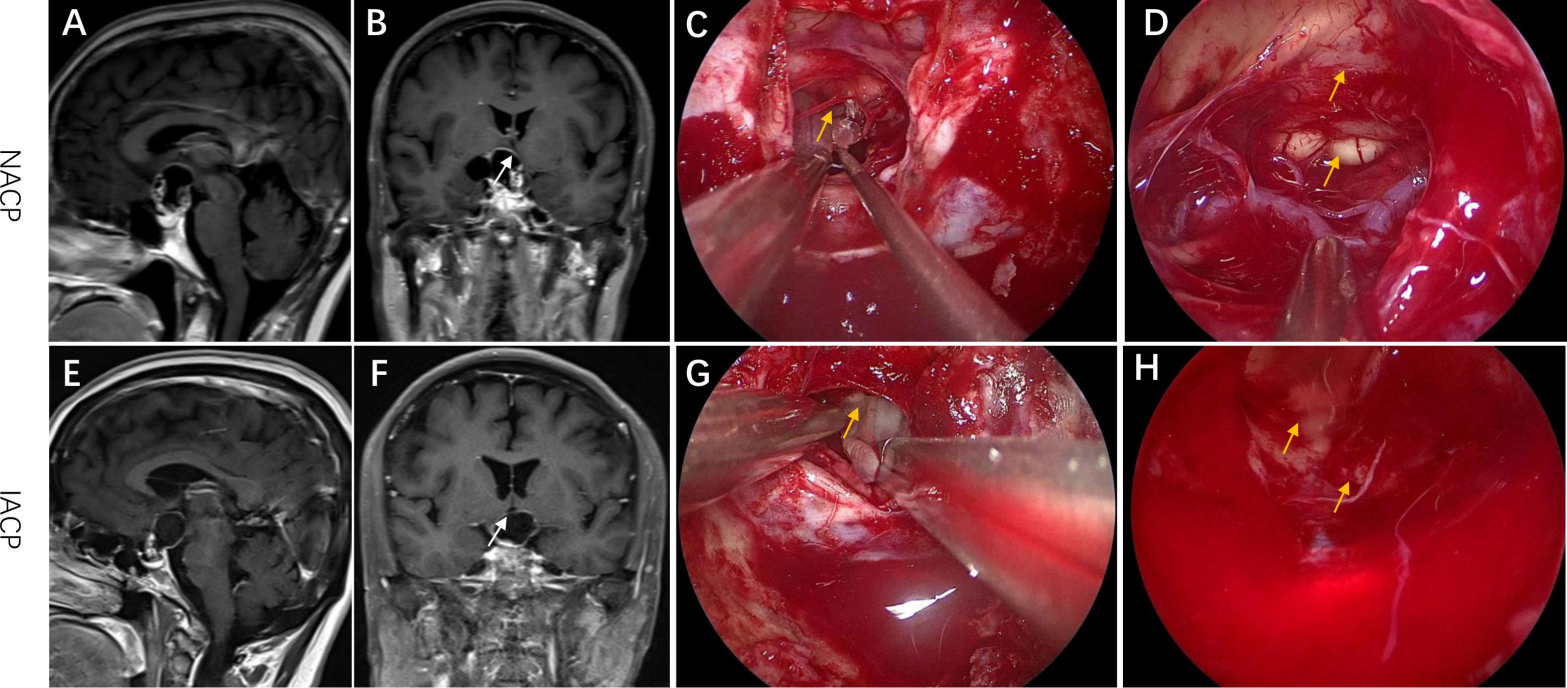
Representative radiological and intraoperative images of IACP and NACP. (A, B) Sagittal and coronal MRI images of NACP. The arrow pointed to the site of TVF. (C, D) The intraoperative images of EEA surgery for NACP. (C) The arrow pointed to the interface between the hypothalamus and the tumor capsule. (D) The arrow indicated the TVF and mammillary bodies. (E, F) Sagittal and coronal MRI images of IACP. The arrow pointed to the site of TVF. (G, H) The intraoperative images of EEA surgery for IACP. (G) The arrow pointed to the connected plane between the hypothalamus and the tumor capsule. (H) The arrow indicated the TVF. EEA, expanded endonasal approach; TVF, third ventricle floor; NACP, non-invasive adamantinomatous craniopharyngioma; IACP, invasive adamantinomatous craniopharyngioma.
